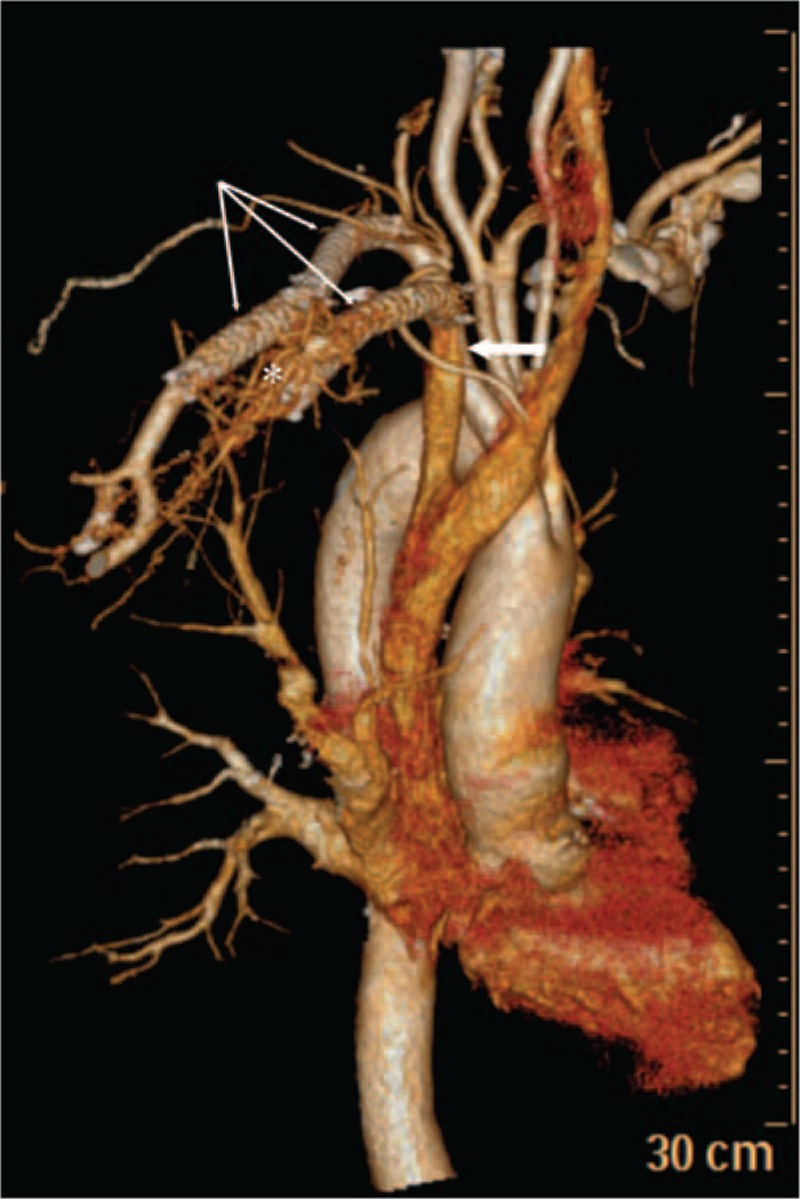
Computed tomographic angiography during 3-month follow-up showed the patent stents in the right subclavian artery and vein (thin arrows), the patent subclavian vein (thick arrow), and an acceptable thrombosis of the multiple arteriovenous malformations (asterisk).
